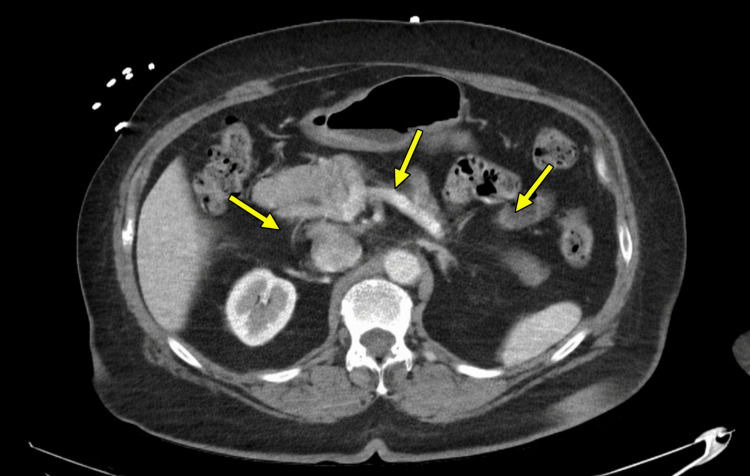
Axial contrast-enhanced CT abdomen, portal venous phase Yellow arrows highlight the pancreatic head/neck region, pancreatic body, and distal pancreatic body/tail region with adjacent peripancreatic fat planes. The pancreas demonstrates preserved enhancement without edema, peripancreatic inflammatory change, fluid collection, necrosis, or pancreatic ductal dilation. No common bile duct dilation is visualized in this image.
